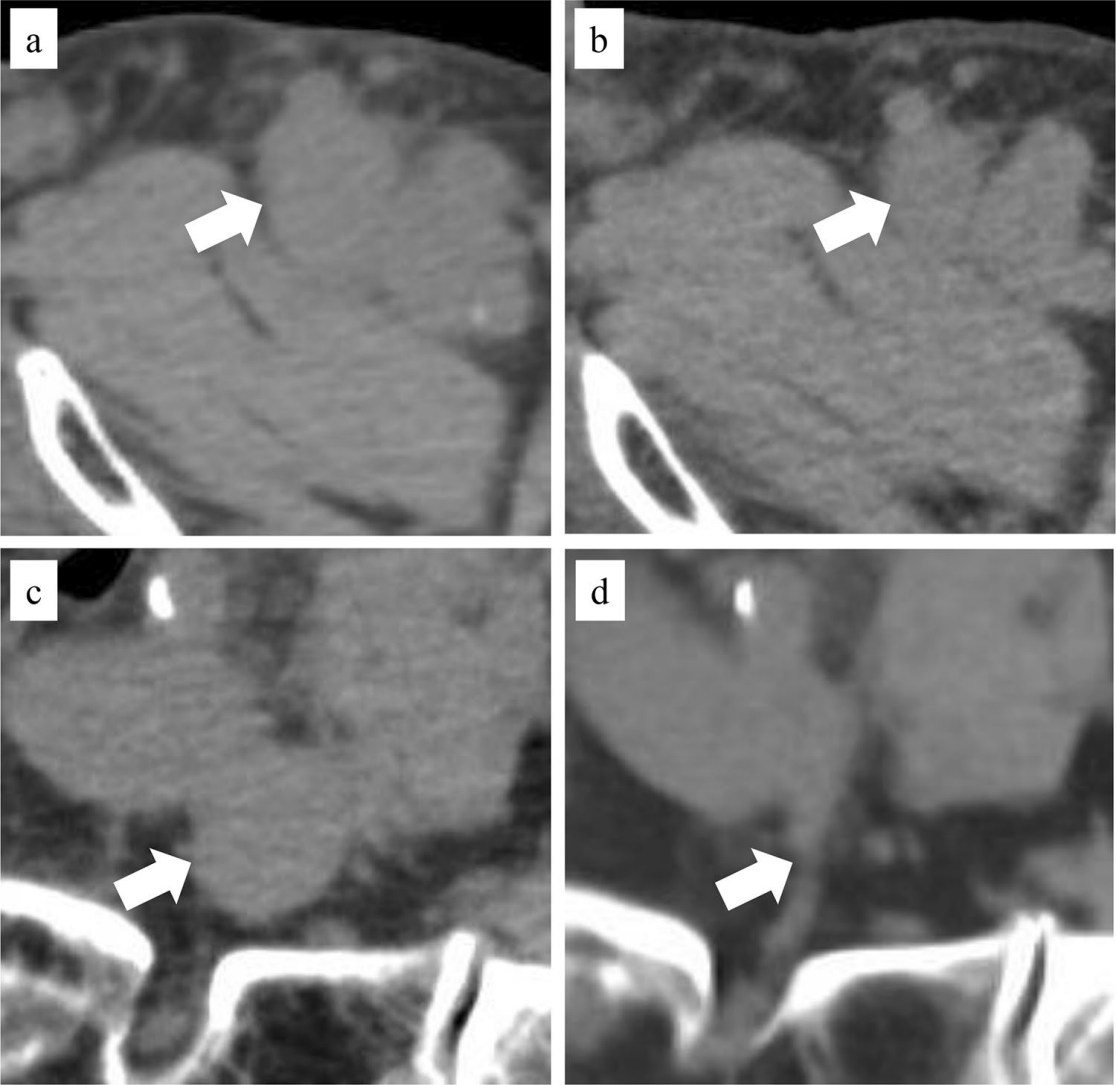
CT image of case 1. a Left inguinal lymph node before radiotherapy; b left inguinal lymph node 2 months after radiotherapy; c left iliac lymph node before radiotherapy (unirradiated assessment lesion); and d left iliac lymph node 4 months after radiotherapy (unirradiated assessment lesion). White arrow, tumour; *CT* computed tomography
